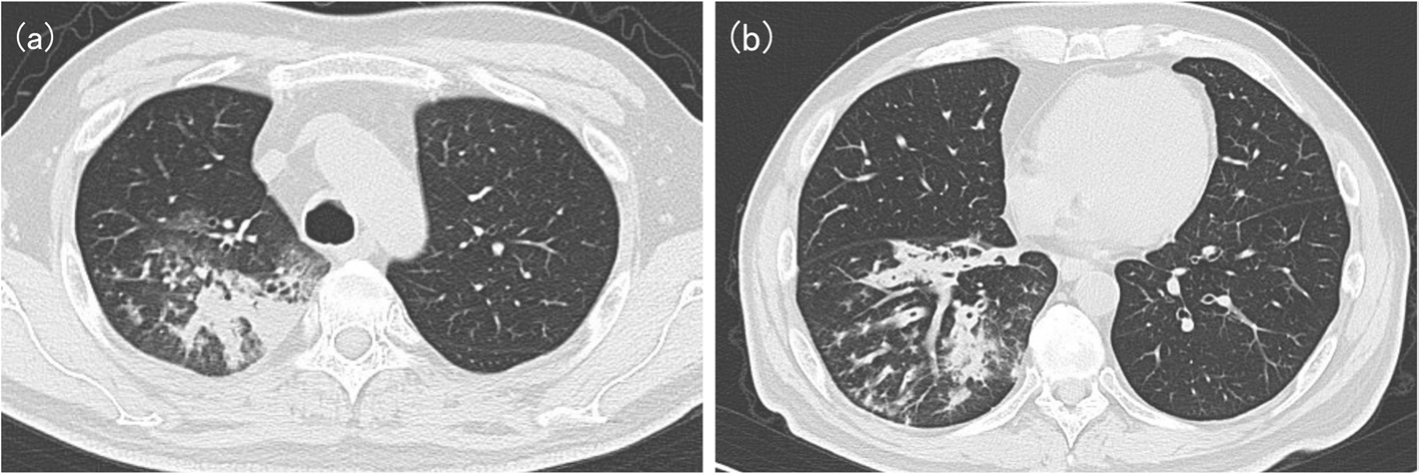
CT showing bronchial wall thickening and focal consolidation at right a upper and b lower lobes
